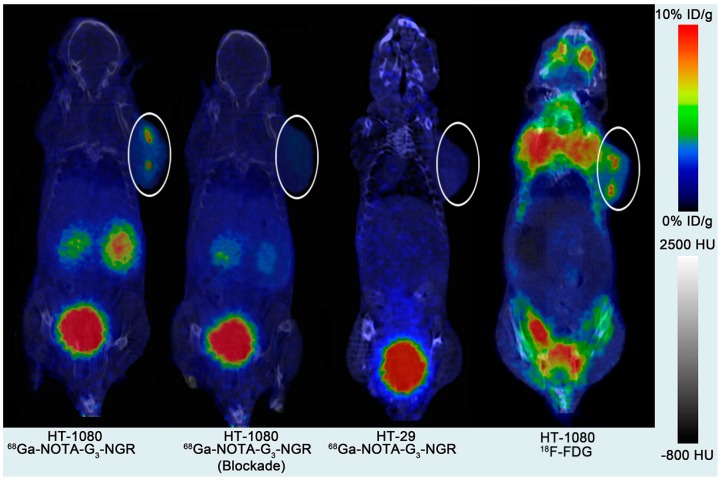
Decay-corrected coronal image slices at 1 h pi of ^68^Ga-NOTA-G_3_-NGR in HT-1080 and HT-29 tumor xenografts with and without co-injection of non-radiolabeled NOTA-G_3_-NGR peptide as a blocking agent. As a comparison, decay-corrected coronal image slice at 1 h pi of ^18^F-FDG in HT-1080 tumor xenografts was also shown. Tumors are indicated using circles.
